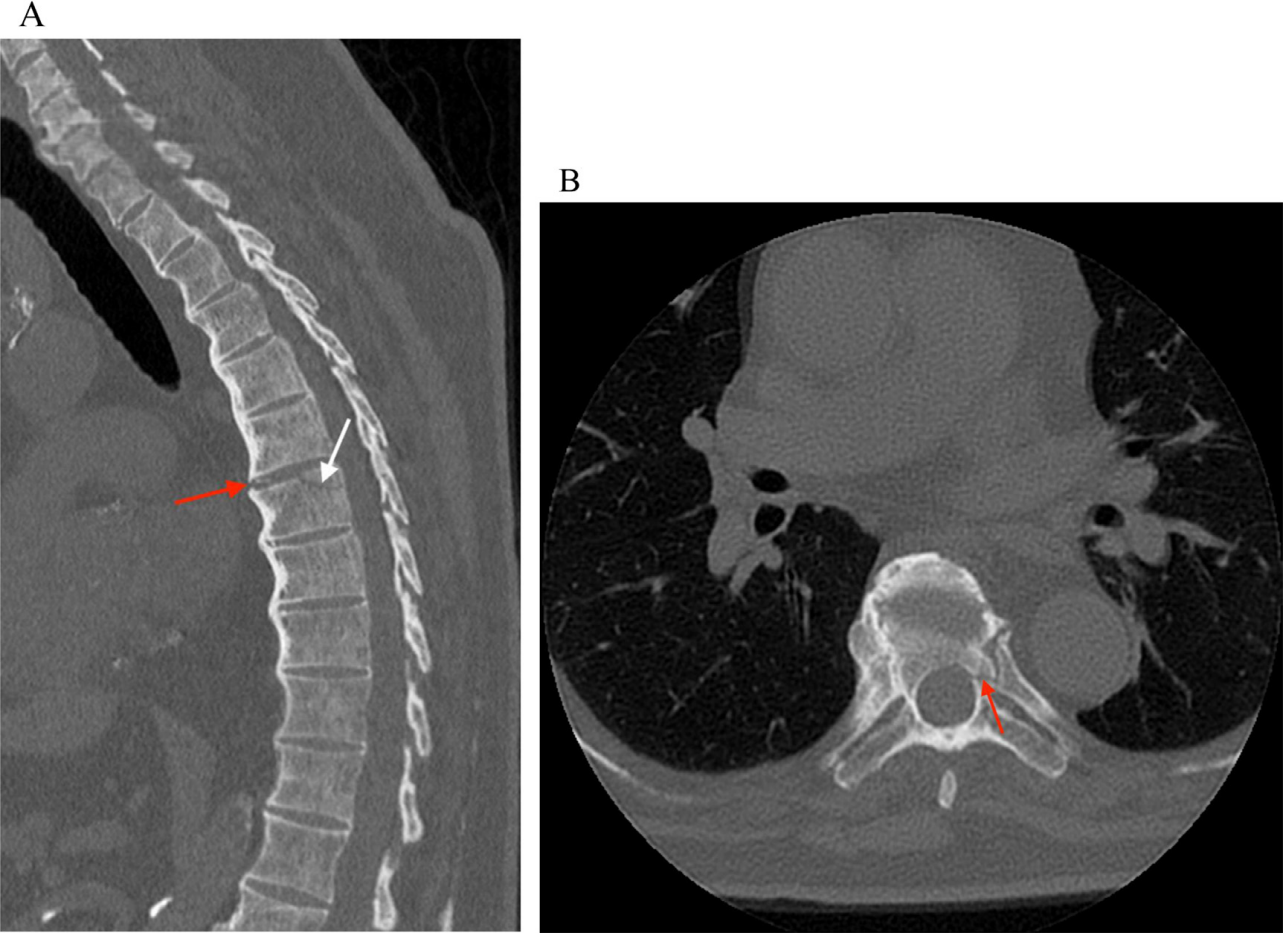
A noncontrast CT image of the thoracic spine. (A) Sagittal CT image demonstrates a nondisplaced oblique fracture of the superior vertebral body of T7 (white arrow). There is widening of the T6-T7 disk space with disruption of the anterior T6-T7 flowing osteophyte (red arrow). (B) Axial CT image demonstrates a nondisplaced linear fracture through the left pedicle of T7 (red arrow).
